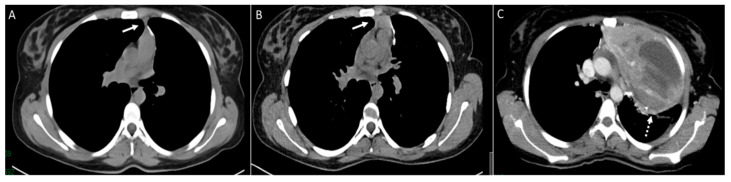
Patient with osteosarcoma and recurrent metastasis post metastasectomy. Axial non-enhanced image (A) shows post-operative changes along the surgical staple line (arrow). Axial non-enhanced image (B) from a follow-up exam shows interval development of mediastinal soft tissue thickening (arrow). Axial enhanced image (C) from a subsequent follow-up CT shows a marked interval enlargement (dashed arrow) representing mediastinal disease recurrence.
